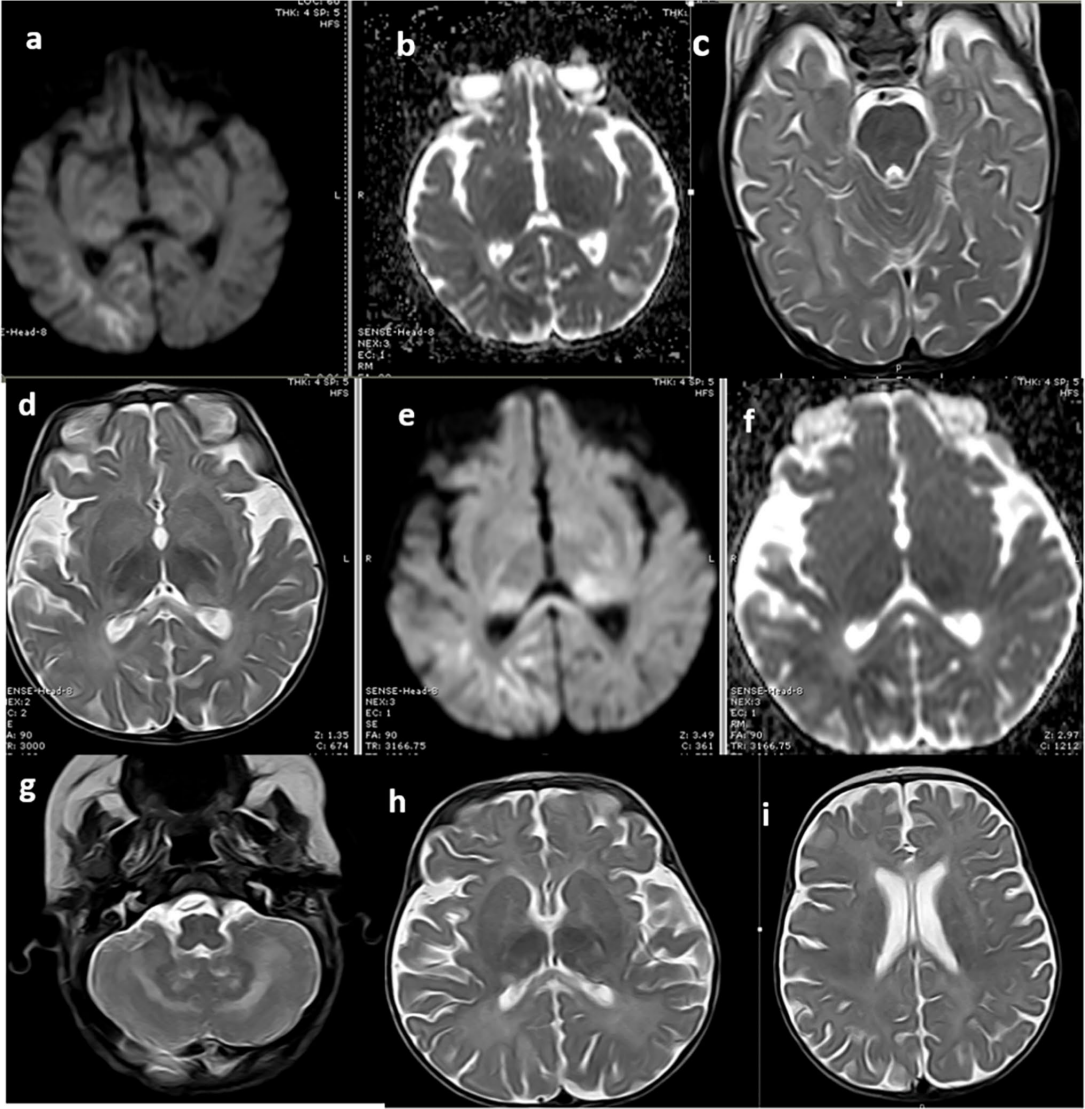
Magnetic resonance imaging manifestations in a female patient with *RAB27A* mutation, presenting with sudden convulsions. a, b, c Axial diffusion-weighted (a), apparent diffusion coefficient (b), and axial T2-weighted (c) images showing right occipital subcortical white matter true diffusion restriction and corresponding high T2 signal intensity. d, e, f Axial T2-weighted (d), diffusion-weighted (e), and apparent diffusion coefficient (f) images showing bilateral asymmetrical posterior thalamic swelling with high T2 signal and true diffusion restriction, more evident on the left side, corresponding with gray matter severity score of 3. g, h, i Axial T2-weighted images at different levels show bilateral symmetrical cerebellar white matter and dentate nuclei patchy high signal in background global atrophic changes, corresponding with a cerebral atrophy severity score of 1, white matter severity score of 3, and total severity score of 7
